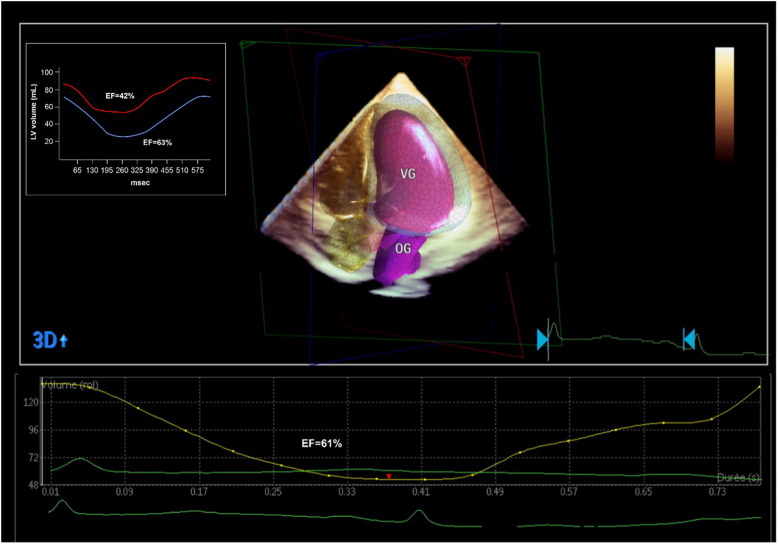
Echocardiographic and PET-CT evaluation of the LVEF after the coronary revascularization. PET-CT showed a significant improvement in LVEF (from 42% to 63%) with a significant decrease globally in LV volumes, denoting an ischemic LV dilatation. 3D echocardiographic evaluation confirmed the normalization of the LVEF.
